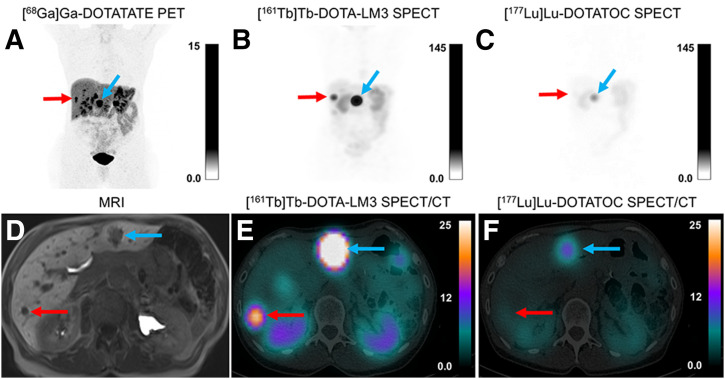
Patient 7 with well-differentiated metastatic NET of pancreas (grade 2, Ki-67 index 5%). (A–F) Baseline maximum-intensity projection of coronal PET/CT 1 h after administration of [^68^Ga]Ga-DOTATATE (A), maximum-intensity projection of coronal SPECT 72 h after administration of 1 GBq of [^161^Tb]Tb-DOTA-LM3 (B) and 72 h after administration of 1 GBq of [^177^Lu]Lu-DOTATOC (C), transaxial T1-weighted baseline MRI (D), and transaxial SPECT/CT 72 h after administration of 1 GBq of [^161^Tb]Tb-DOTA-LM3 (E) and 72 h after administration of 1 GBq of [^177^Lu]Lu-DOTATOC (F). [^161^Tb]Tb-DOTA-LM3 SPECT/CT clearly delineates both liver metastases, despite smaller one measuring only 7 mm (red arrow). In contrast, [^177^Lu]Lu-DOTATOC SPECT/CT visualizes only larger metastasis (blue arrow). Scale bars indicate SUV in A and kilobecquerels in B, C, E, and F.
